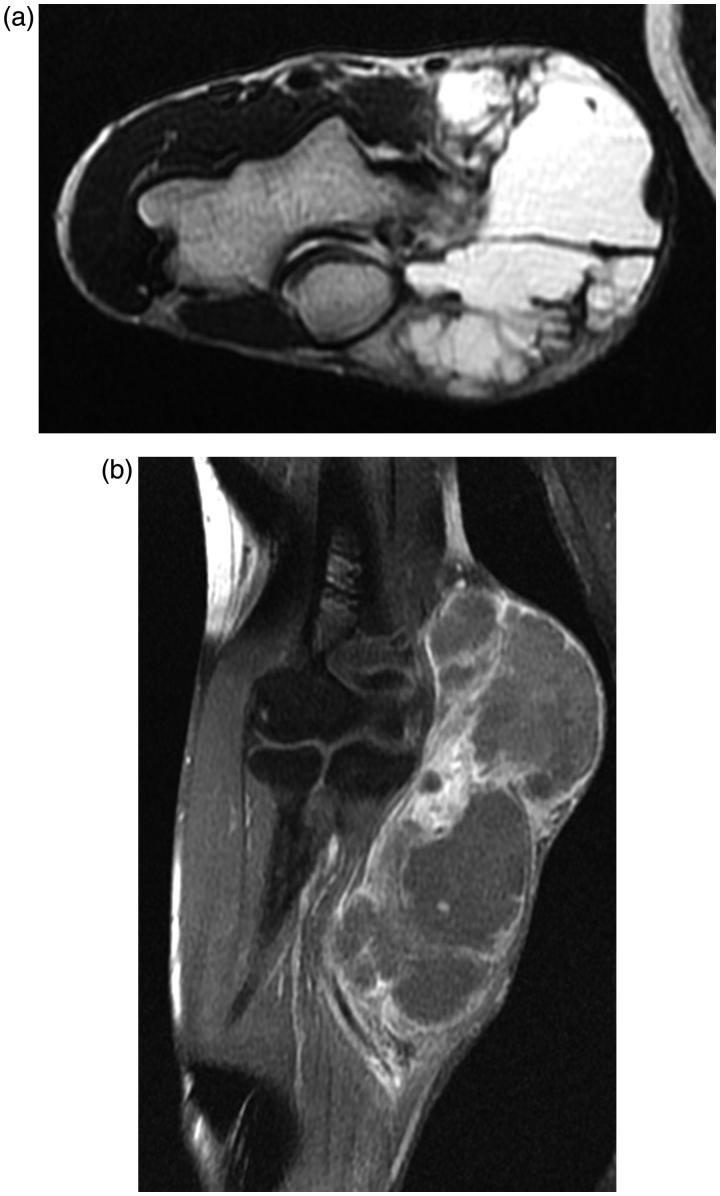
Pre-operative magnetic resonance image, showing the axial T2WI (a), and coronal Gd-DTPA-enhanced T1WI (b).
